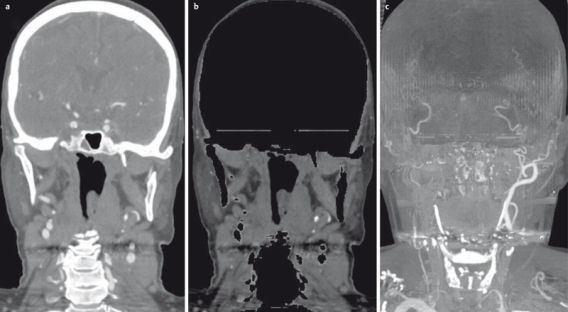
Dual-source CT of the midface. a) Fusion image of the 80 kV and 120 kV datasets. b) Automatic bone removal based on the different attenuation of bone in the two datasets. c) MIP reconstruction of the automatically segmented vessels.
